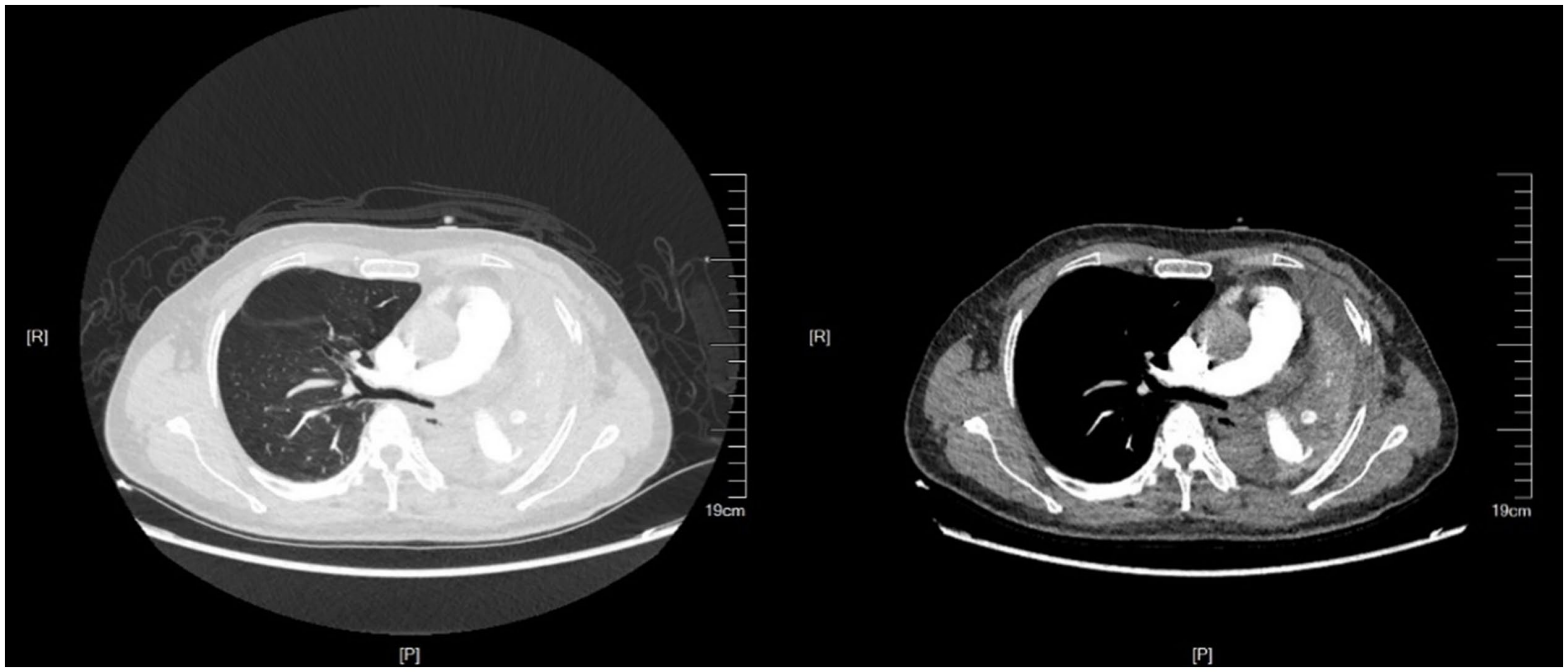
Chest CT at the second visit.
